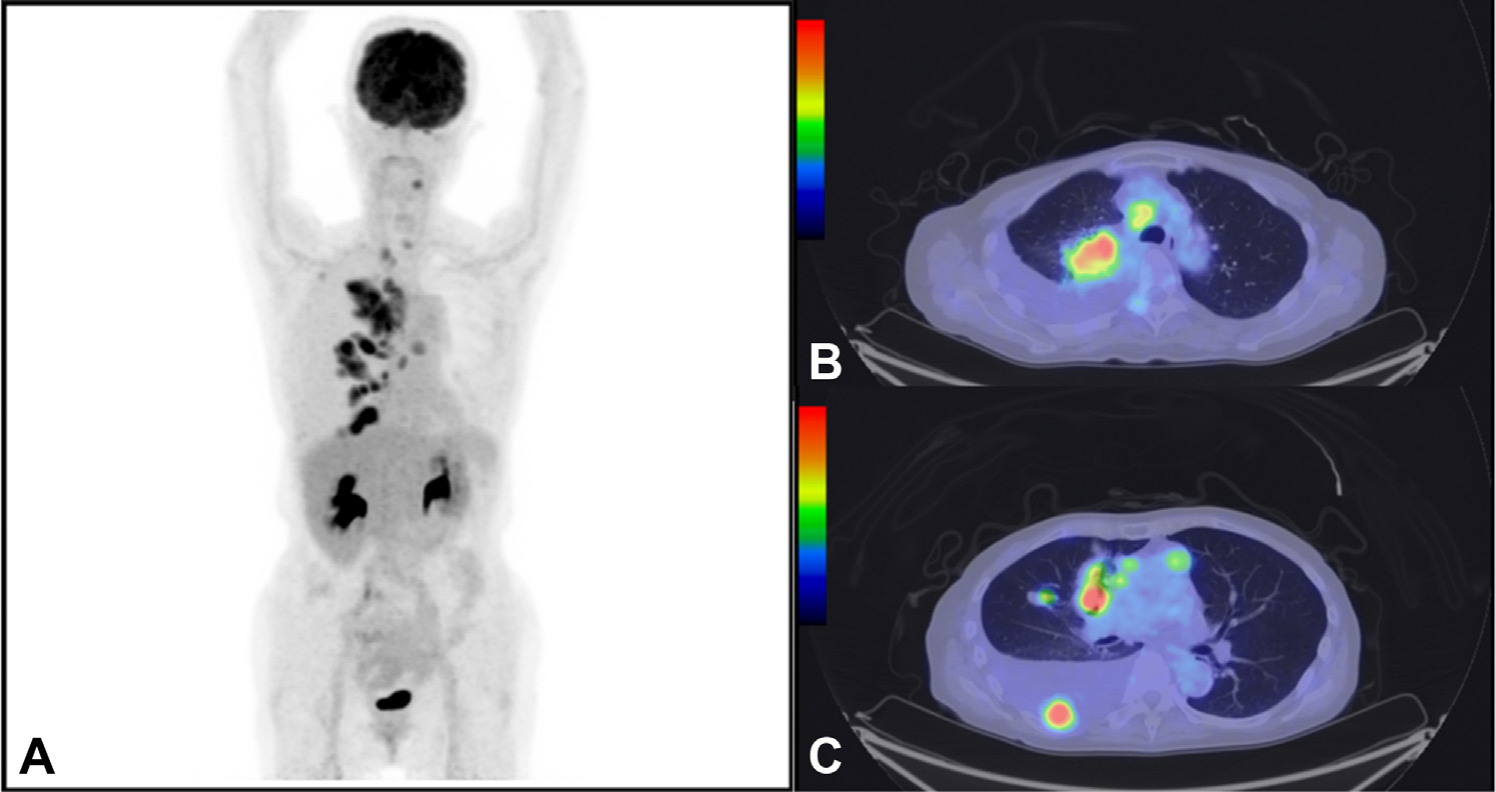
Positron emission tomography-CT on diagnosis. Positron emission tomography-CT revealed multiple lung tumors, pleural effusion, metastatic lesions of mediastinal and neck lymph nodes and bones, and fluorodeoxyglucose accumulation in the same lesions. (*A*) Overall view. (*B*) Tumor at right upper lung and pleural effusion. (*C*) Tumor in the right pleural effusion. CT, computed tomography.
